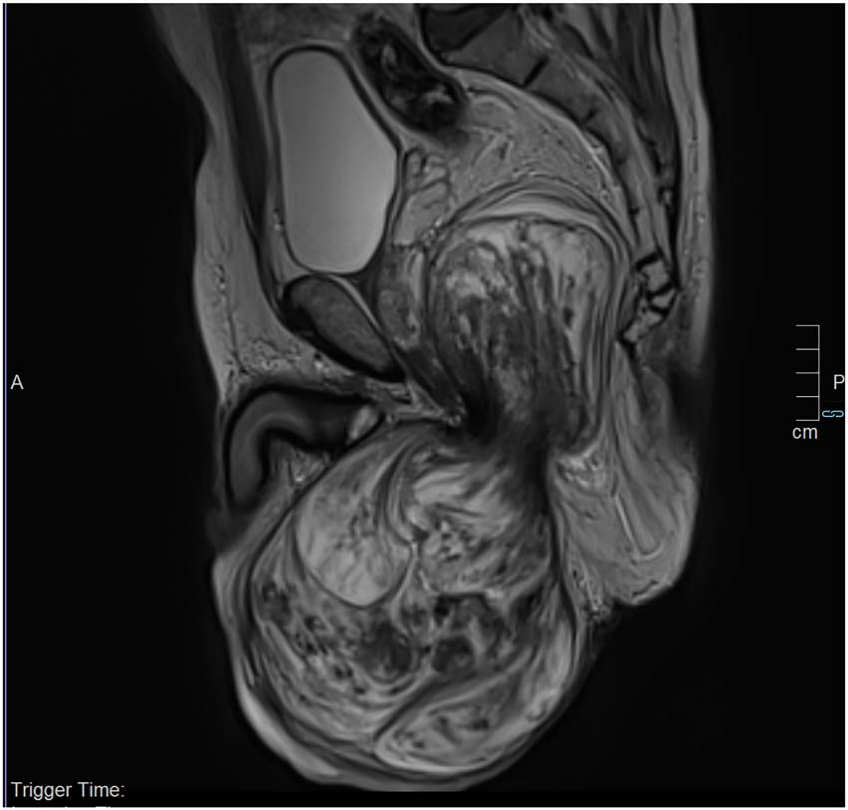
Sagittal MRI section demonstrating clear separation between the base of the penis and the bladder.
